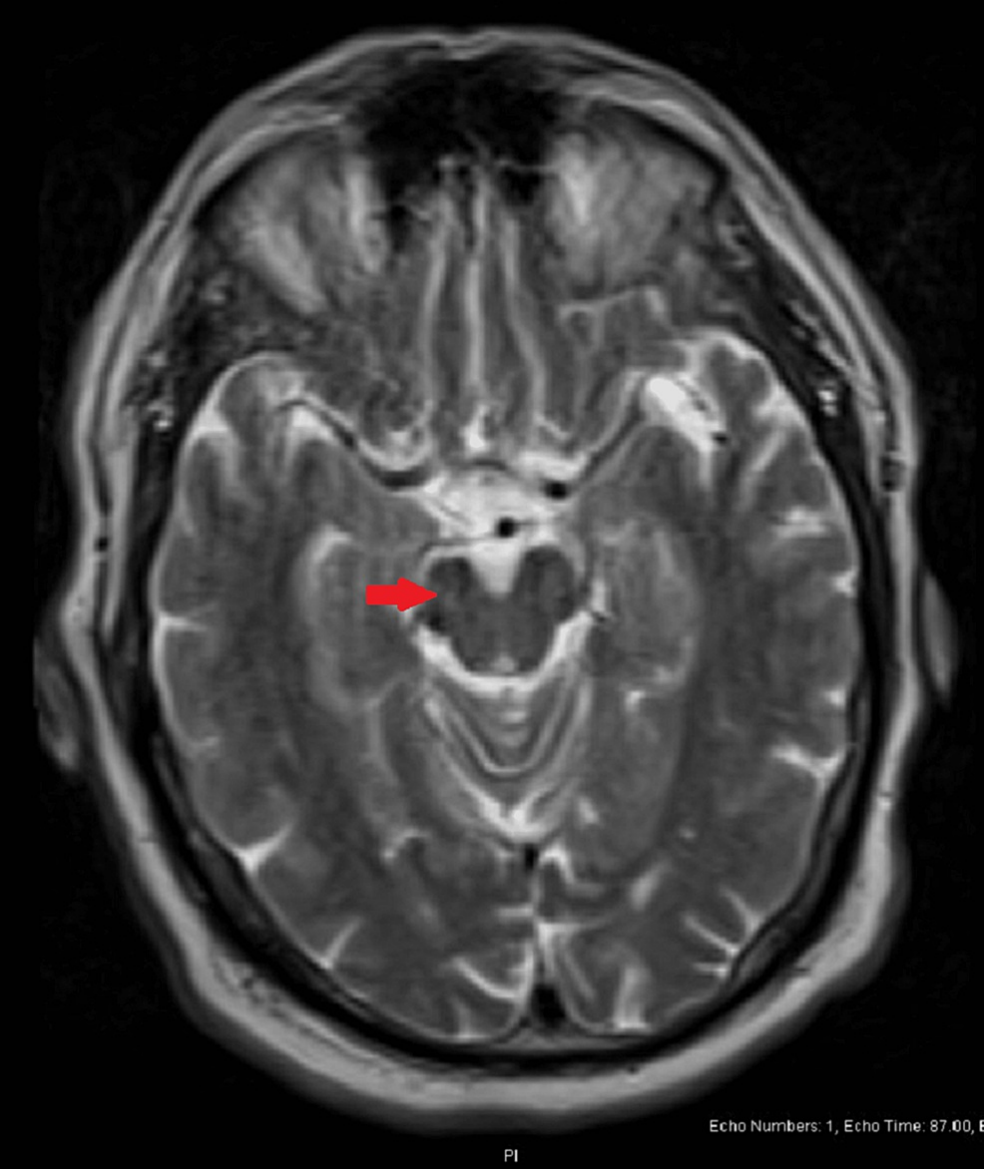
Axial T2-weighted MRI shows right-sided substantia nigra hyperintensity loss (arrow) Image subject to motion degradation MRI = magnetic resonance imaging
